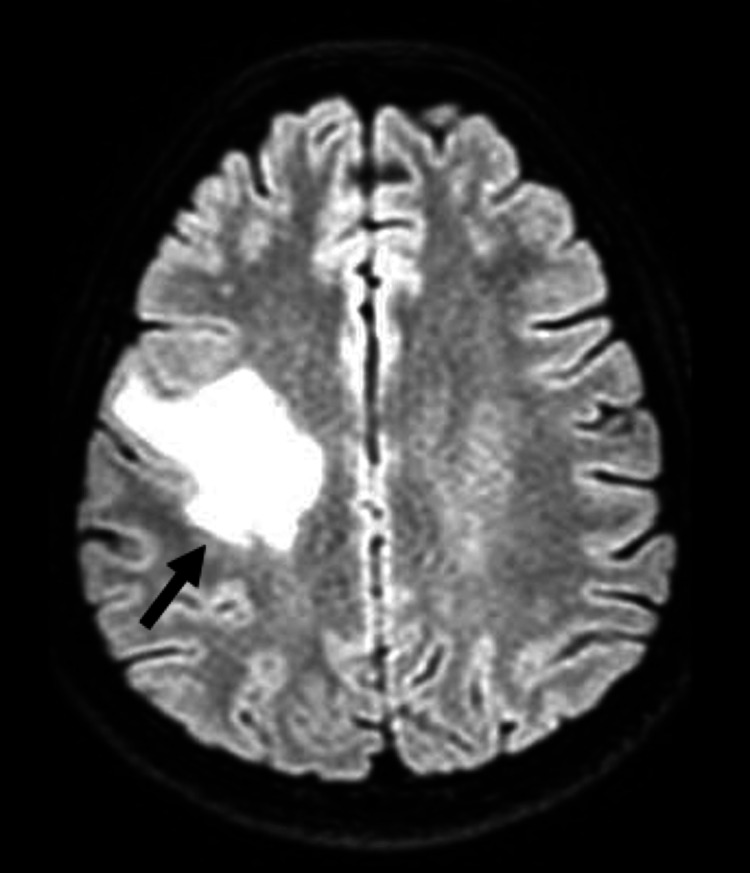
Contrast-enhanced MRI with evidence of lesions suggestive of cerebral tuberculomas (T2-weighted imaging).
